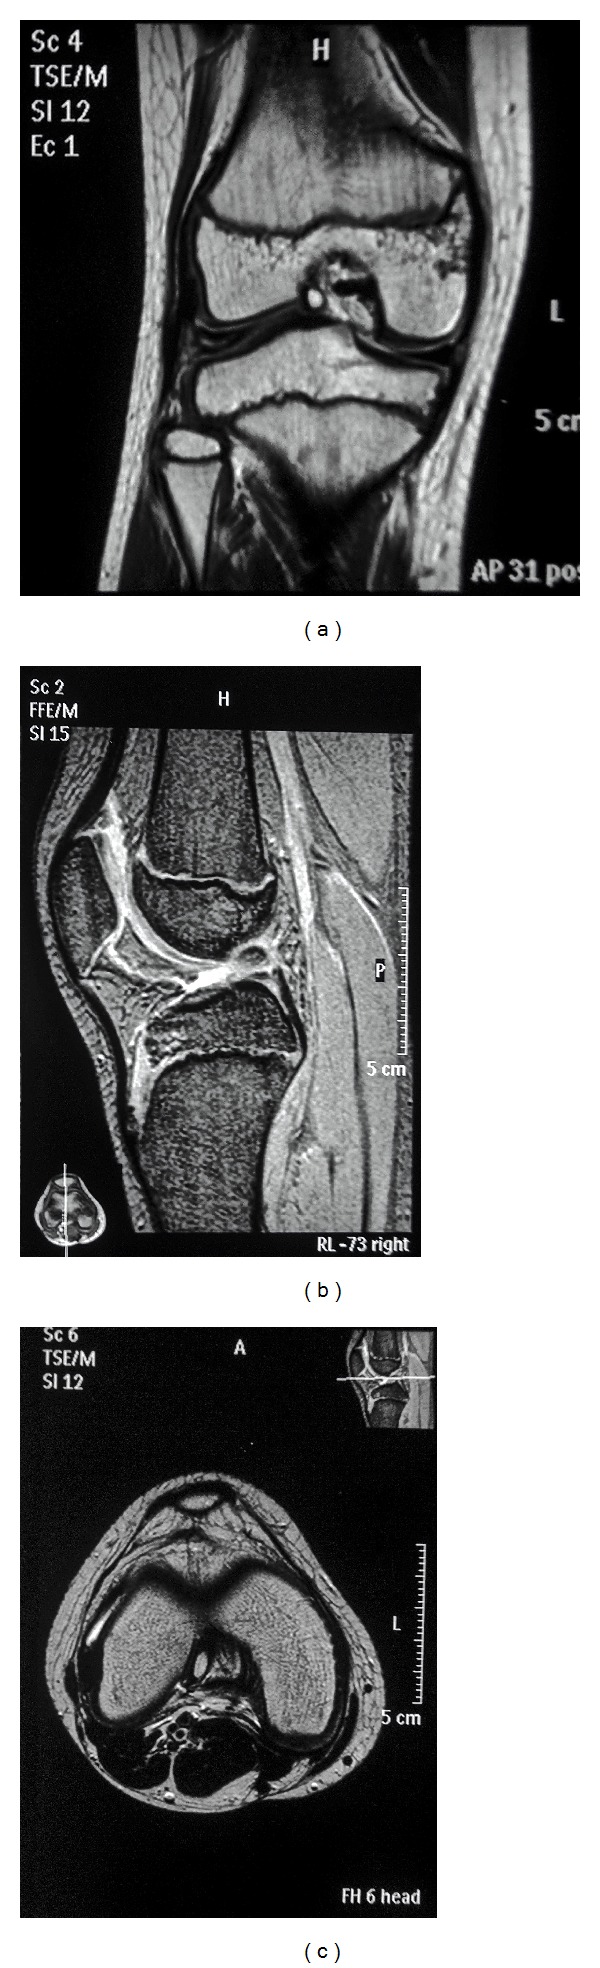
MRI: (a) coronal section, (b) sagittal section, and (c) axial section showing the ossicle within the substance of the anterior cruciate ligament.
